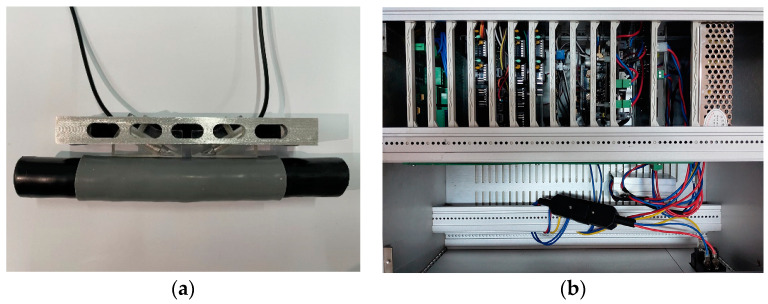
Measurement system for the cold shrink tube stress: (a) ultrasonic probes and (b) FPGA module and data acquisition and transmission module.
